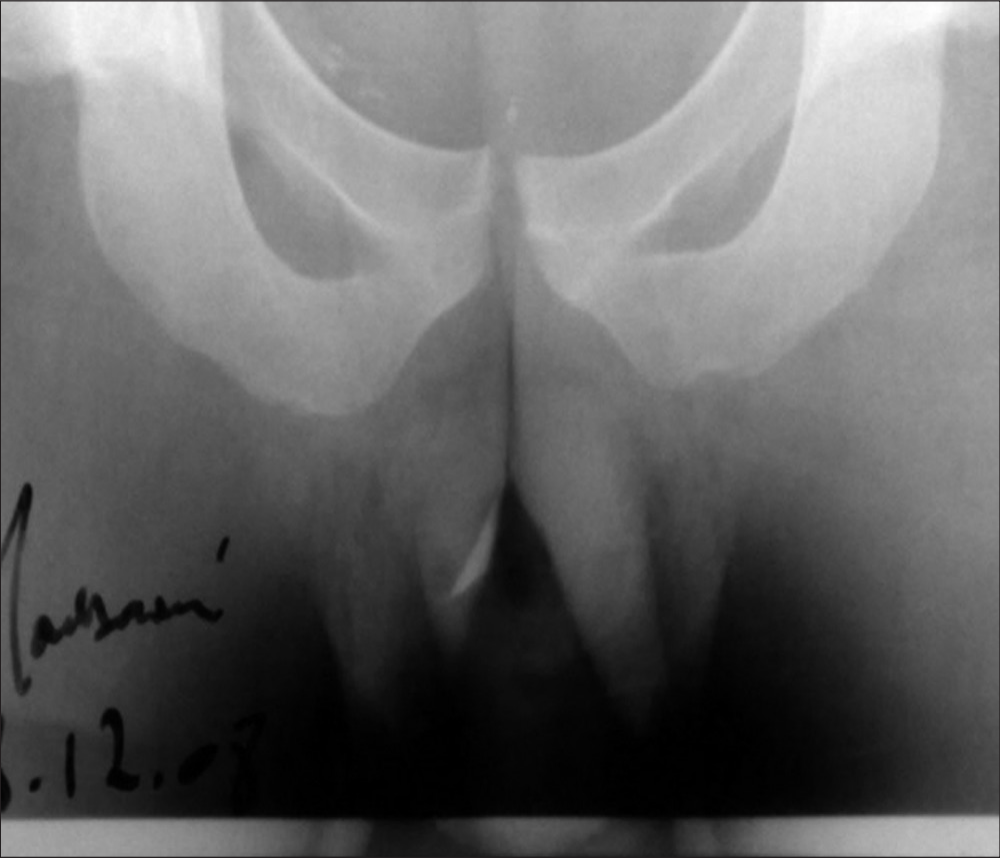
Abdominal X-ray objectified the foreign bodies
